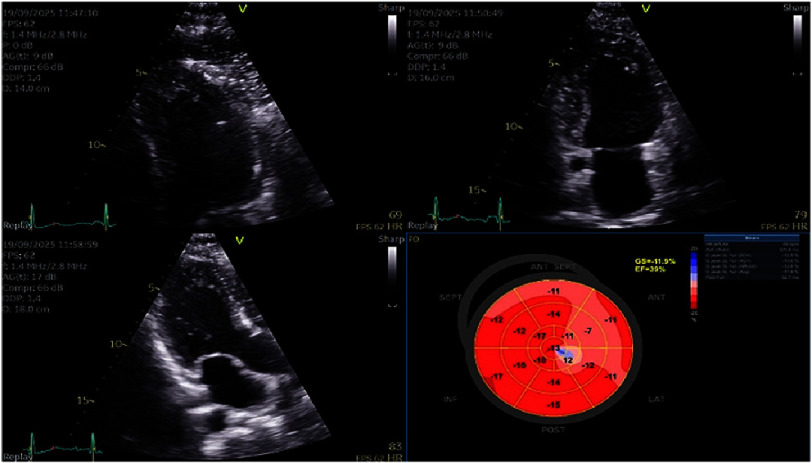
Echocardiographic assessment on day 10 of hospitalization demonstrating LVEF 39% and GLS −11.9%.
